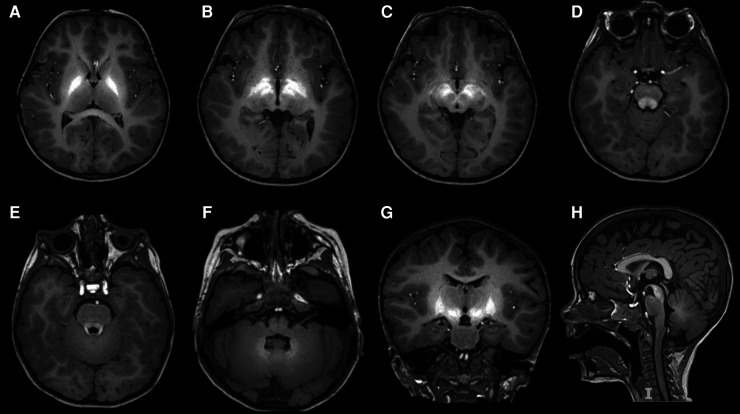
Bilateral and symmetrical hyperintensity in T1-weighted imaging involving globi pallidi (A,B,G), subthalamic nuclei (B,G), substantia nigra (C,H), pontine tegmentum (D), superior cerebellar peduncles (E), dentate nuclei (F), and pituitary gland (E,G), reflecting manganese accumulation.
